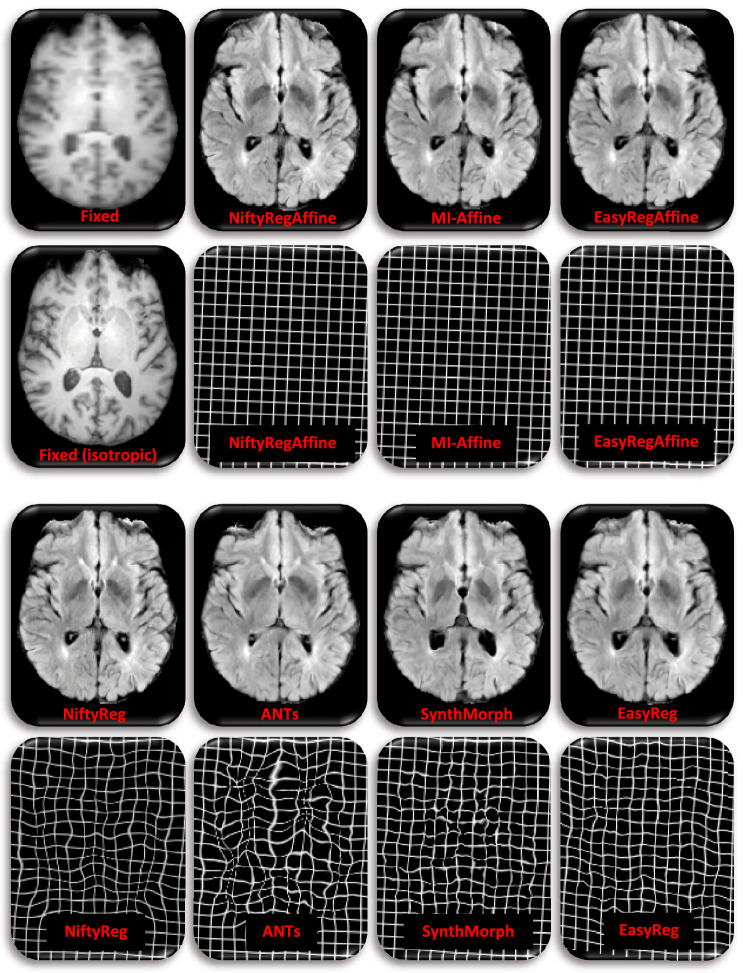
Sample registration from SagT1–AxFLAIR setup, i.e., 5 mm axial FLAIR from ADNI, to 5 mm sagittal T1-weighted scan from IXI. Top left: axial slice of the fixed image and corresponding isotropic slice; the later was *not* used in registration; we display it for easier qualitative assessment of registration accuracy. Rest: corresponding registered slice and deformation field (represented as a deformed grid) for the seven competing methods.
